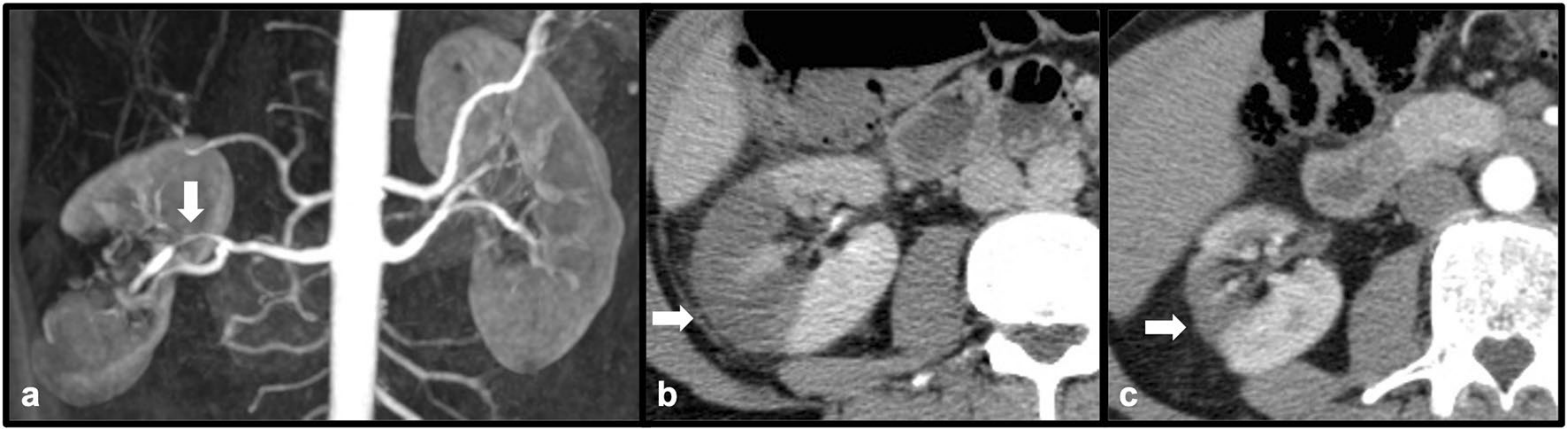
Coronal post-contrast MRI (a) in a patient with Behçet’s disease demonstrates narrowing of a branch of the main renal artery (arrow) with associated cortical defect consistent with infarct. Axial contrast-enhanced CT (b) demonstrates an area of diminished right renal perfusion (arrow) consistent with infarct. Axial contrast-enhanced CT 1 month later (c) demonstrates evolving infarct (arrow) in the right kidney
